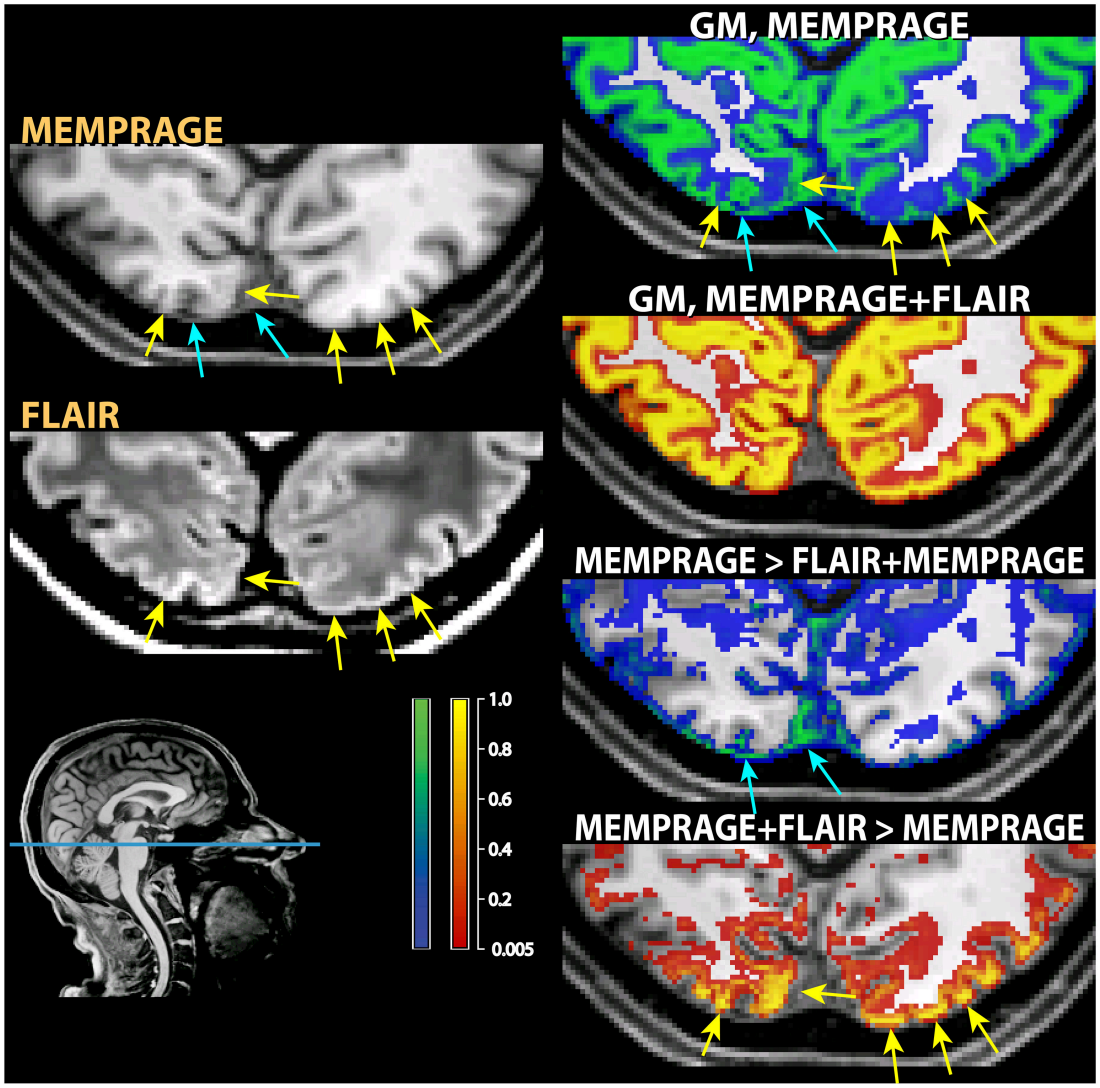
Example of classification differences in the occipital cortex. As in those figures, the segmentation of the T1-weighted images alone is shown as a blue-green overlay, and the multimodal segmentation with the added FLAIR images in red-orange. In the left column the input MPRAGE and FLAIR image are shown. The yellow arrows show loss of contrast in the T1-weighted MPRAGE images, which was retained in the FLAIR images. The right column show the classification outcome in the MPRAGE alone segmentation (top, in green) and in the MPRAGE+FLAIR combination (in red-yellow). The yellow arrows pointing to loss of contrast show in the MPRAGE alone segmentation that these areas achieved a classification of about 0.1–0.2 as gray matter, but 0.9 or more in the combined MPRAGE+FLAIR combination. The images in the bottom right corner show the difference in the classification outcome. Note that the parts adjacent to the cortex where the MPRAGE alone segmentation attributed a higher classification probability than the MPRAGE+FLAIR combination (blue arrows) were not gray matter, but connective tissue. This is apparent from the inspection of the input MPRAGE image, where the dura is evident as a continuous sheet running over the left half of the figure, isointense to gray matter in the MPRAGE data but giving low signal in the FLAIR image.
